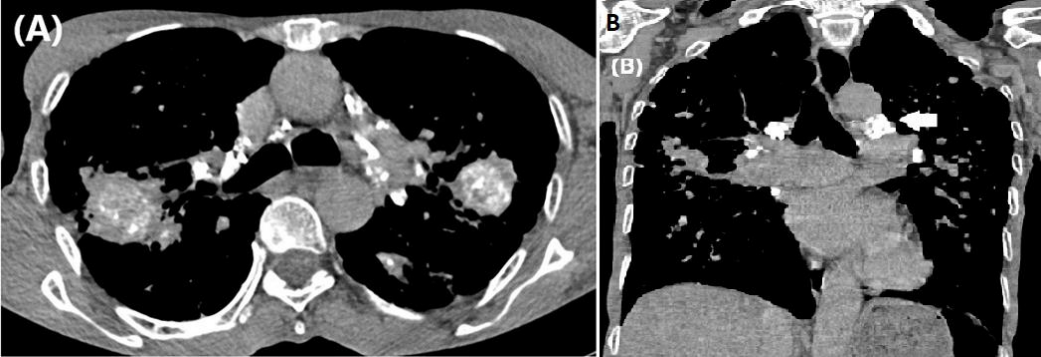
chest computed tomography scan in mediastinal window on axial (A) and coronal (B) views showing the presence of multiple lymphadenopathies involving all territories, most notably the aortopulmonary window (arrow), with peripheral calcifications forming an “egg-shell“ pattern
